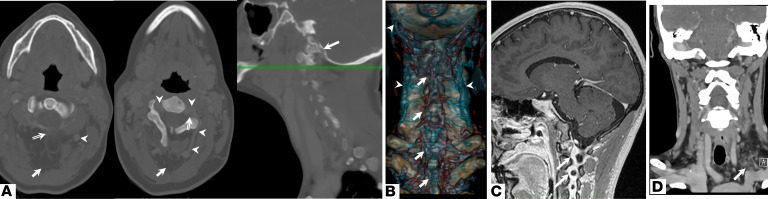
Extrinsic cervical spine venous malformations in HIF-2α gain-of-function syndrome patients. Representative images are shown. (A) Axial CT of the neck with contrast of patient 1 shows cervical dysraphism at the level of C2 (left) due to a subfascial cavernous malformation of the upper neck (arrow). The malformation is continuous with the epidural veins (double-lined arrow) and large veins of the external spinal system (arrowhead). At the level of C3–4 (middle), the malformation (arrow) drains to a persistent segmental vein (arrowheads) of the spinal column bilaterally. These surround the vertebral artery (double-lined arrow). Large veins are seen throughout the soft tissues. Sagittal view of the left side of the same CT of the neck (right) showing the corresponding axial plane (green line). The posterior condylar emissary vein (arrow) is also seen draining to the malformation. (B) Posterior coronal view of the volumetric reconstruction of CT of the neck with contrast of patient 1 highlighting contrast and bone and excluding soft tissue demonstrates the veins (blue; arrowheads) entering and within the cavernous malformation (arrow) lined by persistent mesenchyme (red outline) extending from beneath the skull base down the length of the cervical spine. (C) Sagittal T1-weighted postcontrast MRI of the head demonstrates large sinusoidal veins (arrows) throughout the left lateral aspect of the neck of patient 2. (D) Coronal CT of the neck with contrast of patient 1 shows a venous malformation draining to the left jugular and subclavian veins (arrow).
